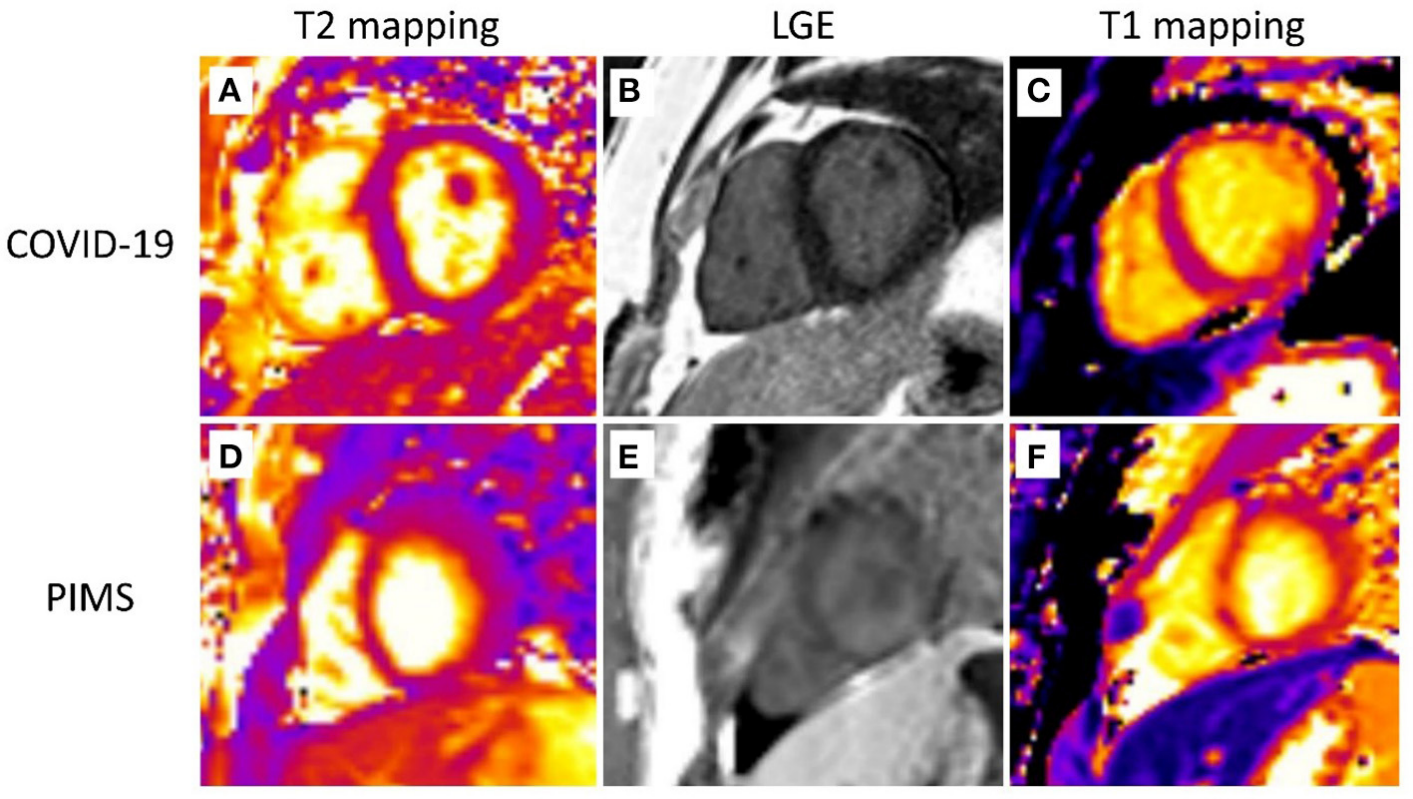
Cardiovascular magnetic resonance. Representative cardiovascular magnetic resonance images are shown. The first row depicts a 15-year-old patient after severe COVID-19 infection. T2 mapping (A), LGE (B), and T1 mapping (C) images display subepicardial and intramural LGE and prolonged T1 relaxation (up to 1,200 ms) along the anterior and anterolateral free wall of the LV with no signs of myocardial edema (normal T2 relaxation time-−45–47 ms). The second row shows images of an 11-year-old patient with pediatric multiorgan inflammatory syndrome (PIMS). T2 mapping (D), LGE (E), and T1 mapping (F) images display similar changes (subepicardial and intramural LGE and prolonged T1 relaxation up to 1,180 ms with normal T2 relaxation time) with a slightly different distribution—along the lateral and posterolateral free wall of the LV.
